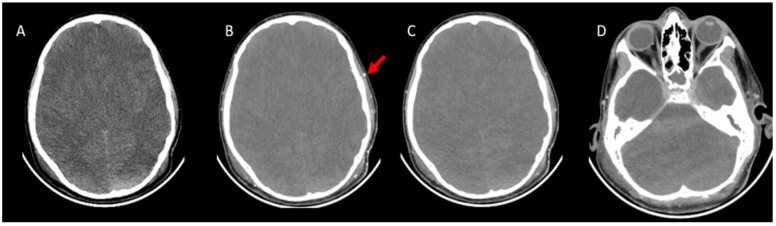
CTA images were taken with the brain death protocol of a 13-year-old boy who was brain dead. (A), Axial pre-contrast CT image shows cerebral edema with the disappearance of the ventricles and flattening of the subarachnoid sulci. (B), Axial post-contrast arterial-phase (20th second) image shows opacification of superficial temporal artery (red arrow), which indicates adequate technique. (C), Axial post-contrast venous-phase image (60th second). The arterial phase and venous phase show an absence of opacification of the bilateral MCA and branches. (D), The venous phase shows the absence of opacification of the ICV and SPV. CTA: CT angiography, MCA: Middle cerebral artery, ICV: Internal cerebral vein, SPV: Superior petrosal vein.
